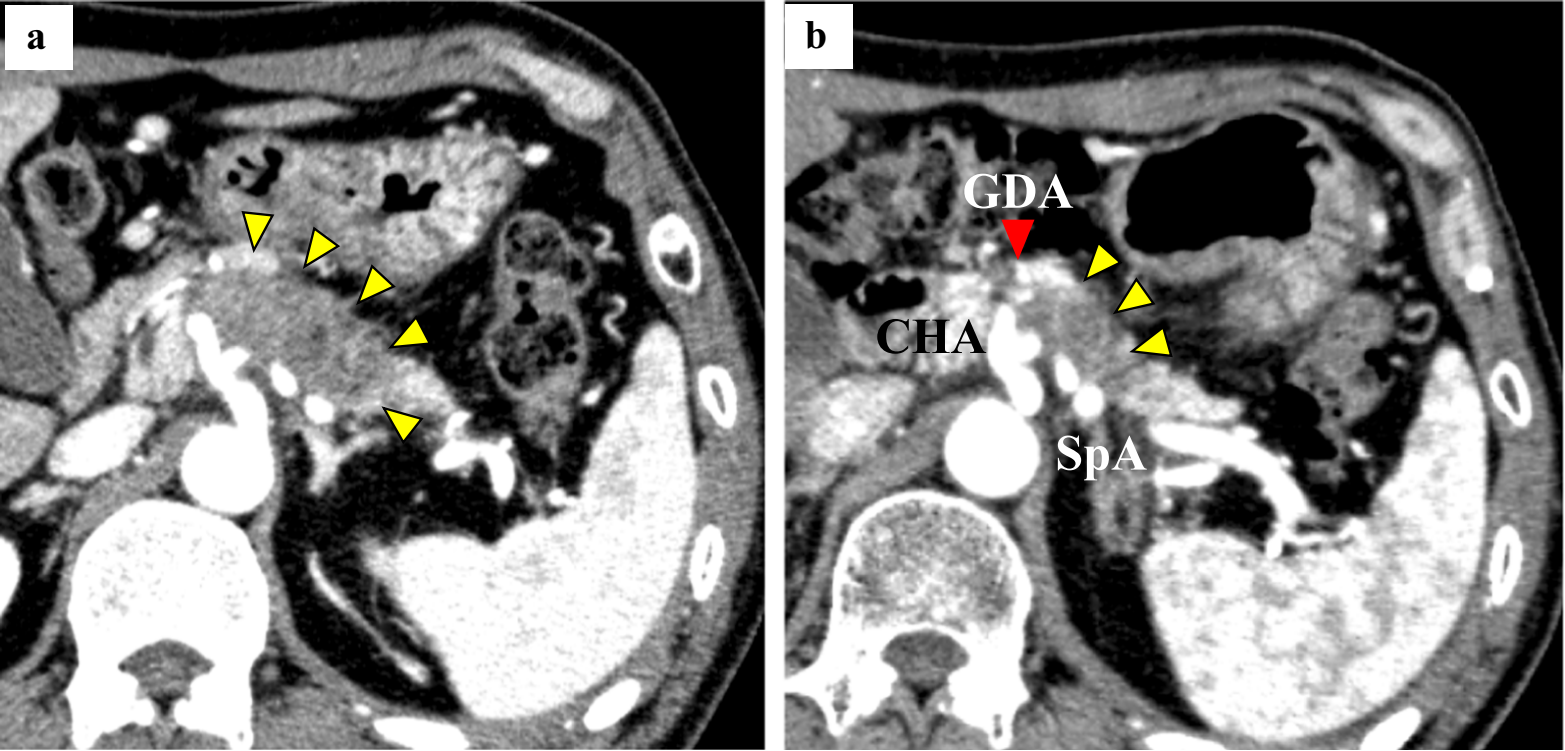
Abdominal CT changes before and after chemoradiotherapy. a Yellow arrows indicate the pancreatic tumor before chemoradiotherapy, and the red arrow indicates GDA. b After chemoradiotherapy, the size of the main tumor was reduced from 54 to 31 mm, but abnormal soft tissue shadows around main arteries were still detected
